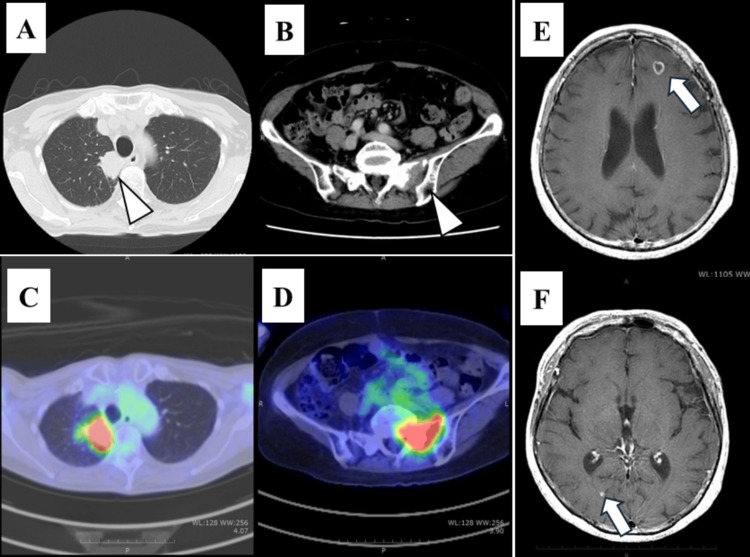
Radiological findings of the tumor at the initial examination (A) CT findings. A mass is seen in the upper lobe of the right lung (white arrowheads). (B) CT findings. Osteolytic changes associated with bone metastasis were observed in the sacrum near the sacroiliac joint. (C) ^18^F-FDG-PET/CT image showing that fluorodeoxyglucose uptake was increased in the mass in the upper lobe of the right lung. (D) ^18^F-FDG-PET/CT image showing that fluorodeoxyglucose uptake was increased in the sacroiliac joint area of the sacrum. (E,F) Contrast-enhanced magnetic resonance imaging of the brain shows enhanced nodules in the left subfrontal cortex and right occipital lobe (white arrows) ^18^F-FDG-PET/CT: ^18^F-2-fluoro-2-deoxy-D-glucose positron-emission tomography/computed tomography
